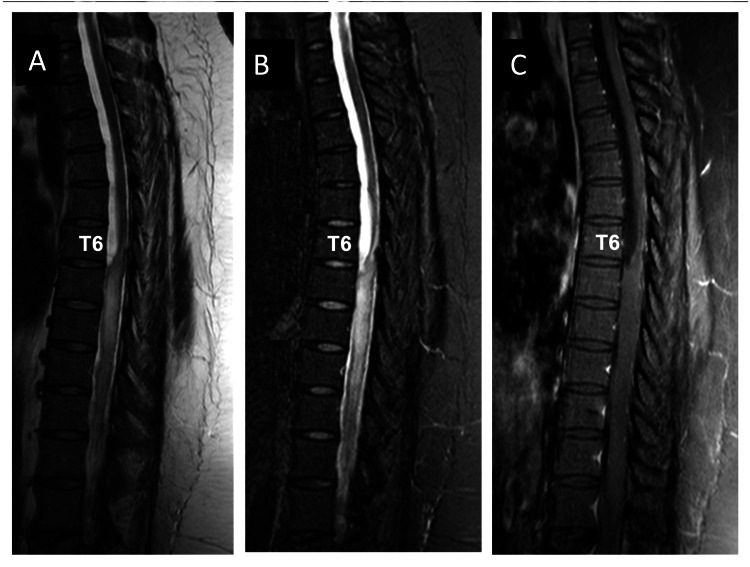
Sagittal T2-weighted (A), T2-STIR (B), and T1-weighted (C) MRI, one month before the first laminectomy, show a reduction of spinal cord edema (T7-T9 levels) and an increase in the cystic mass to the T5-T6 levels.
